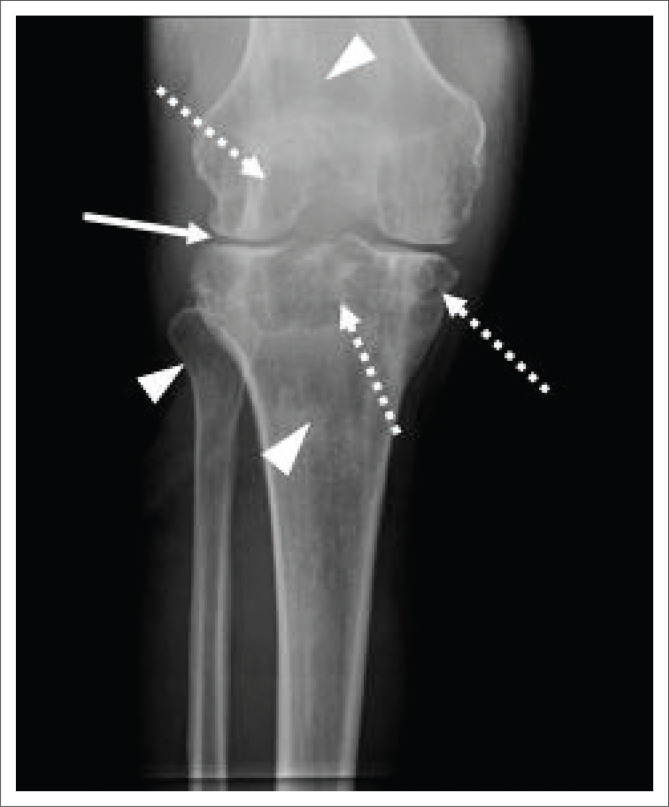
A 41-year-old male, with human immunodeficiency virus status unknown, presented with a 2-year history of chronic right knee pain and a draining sinus. Erythrocyte sedimentation rate at the initial visit was raised. Conventional radiograph of the knee demonstrates joint space narrowing (solid white arrow), subchondral cyst formation (white arrows with dotted lines) and juxta-articular osteopenia (white arrow heads). The constellation of findings is known as the Phemister triad.
